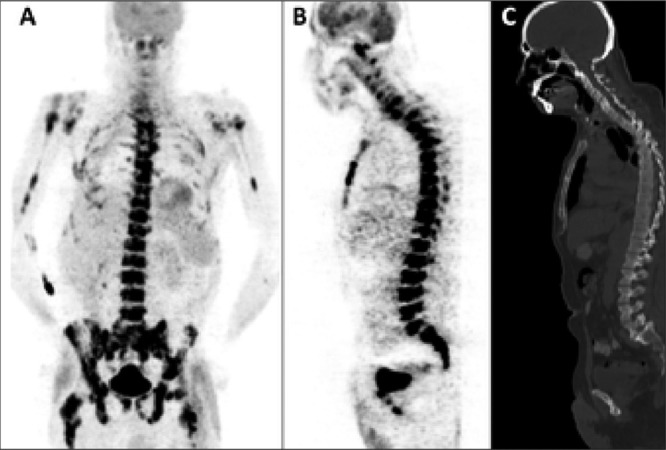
PET images of anterior MIP (A), sagittal PET (B) and CT(C) showinvolvement of bone marrow in several anatomical parts, the most prominentones being vertebral column, pelvic bones, and proximal femur. Bonemarrow biopsy revealed large B cell lymphoma
